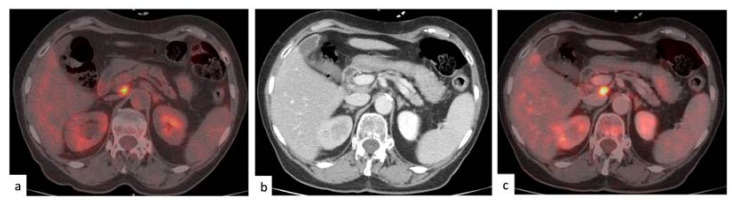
Concordant findings on positron emission tomography/low-dose computed tomography (PET/ldCT) and PET/contrast enhanced (ce)CT. An inter-aortocaval lymph node, with an intensive focal fluorodeoxyglucose (FDG) uptake, is noted on the PET/ldCT (a) on the ceCT part (b) and the PET/ceCT (c). Most lymph node and peritoneal lesions were classified concordantly by the two imaging methods.
